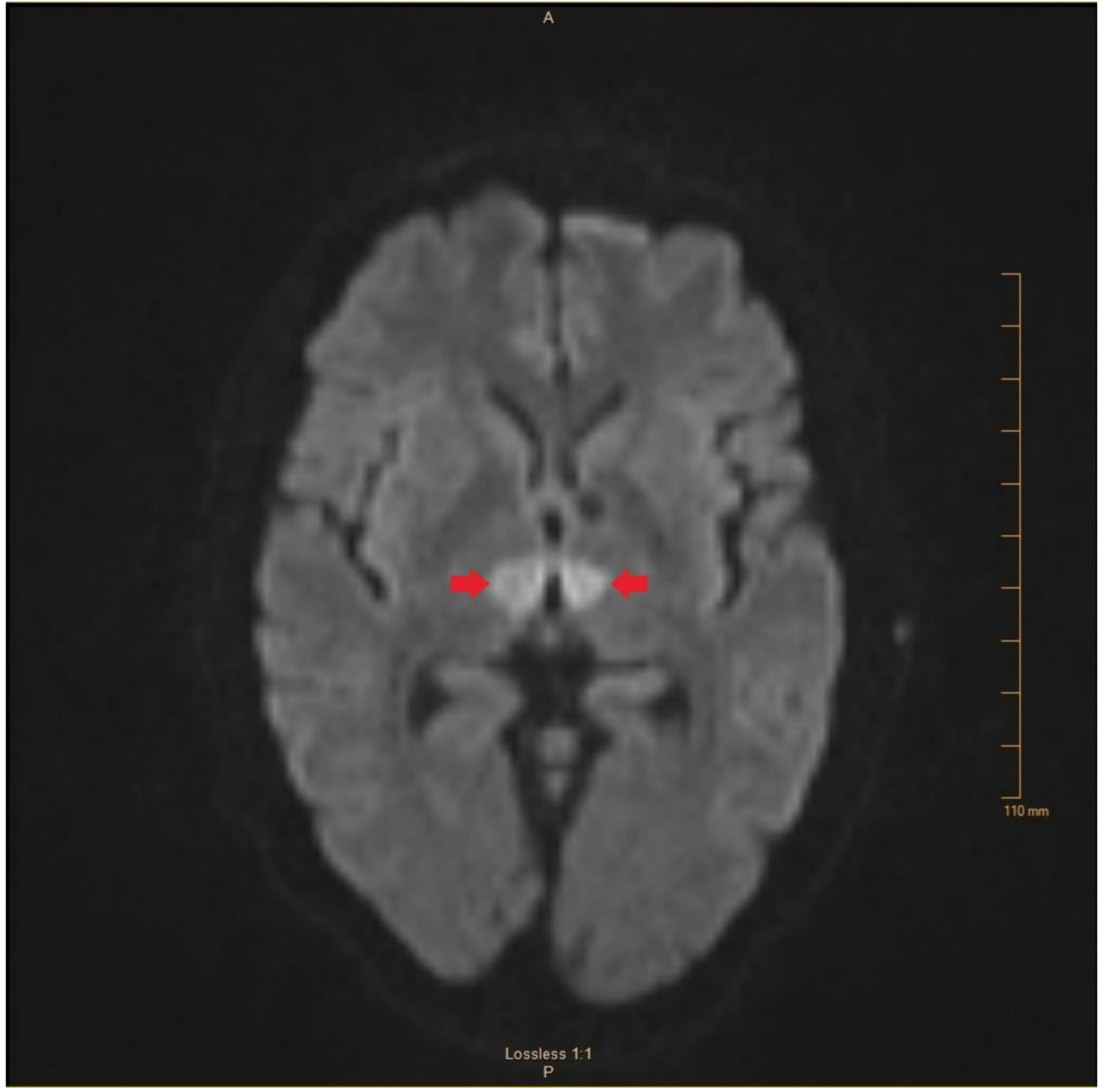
Diffusion-weighted MRI of the brain demonstrating increased signal in the distribution of the AOP. MRI, magnetic resonance imaging; AOP, artery of Percheron.
